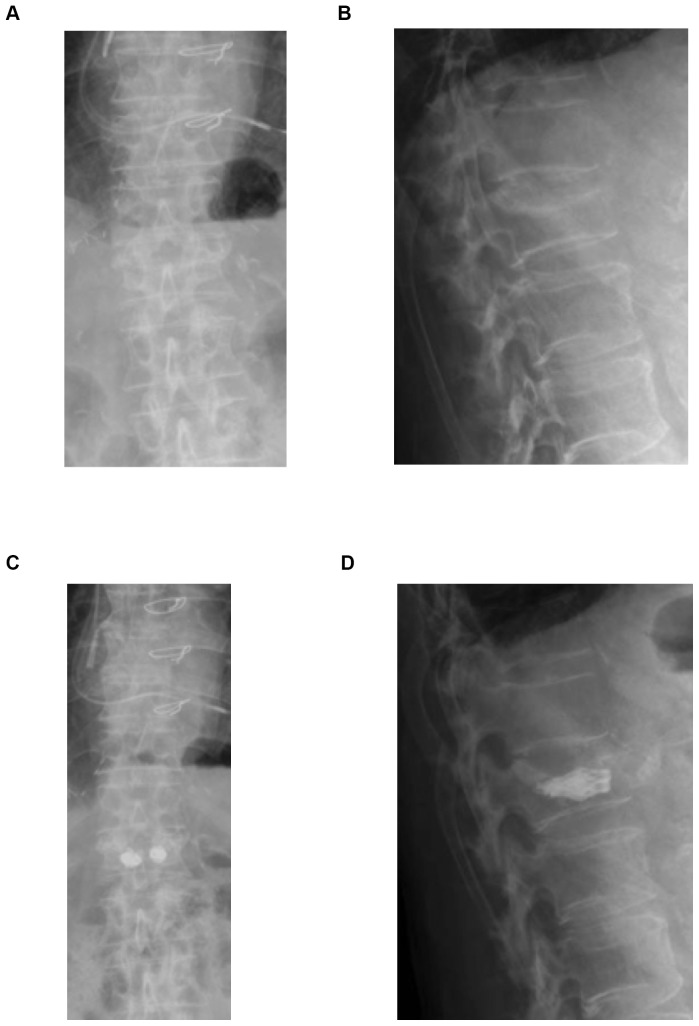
Case presentation with Osseofix® system. (a–d): Radiological case report. Preoperative (a, b) and postoperative (c, d) radiograph of L1 compression fracture and stabilization with Osseofix® system.
